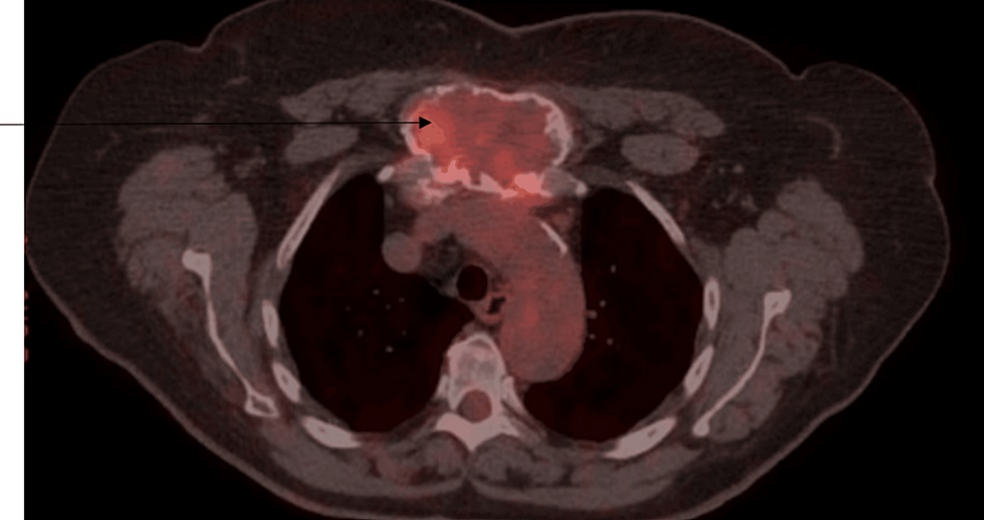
PET/CT showing the manubrial expansible lesion. The arrow points to the increased uptake in the expansile lesion in the manubrium on the PET scan. PET/CT = positron emission tomography/computed tomography
